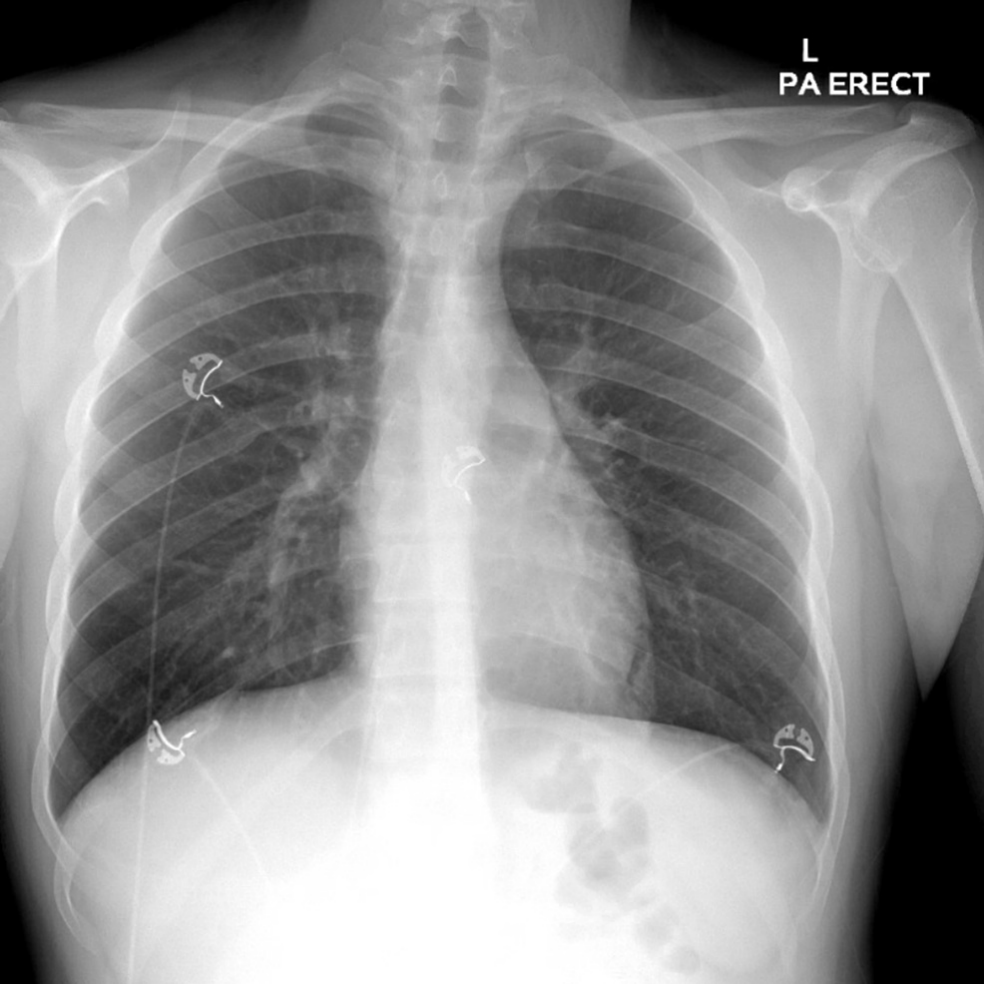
Chest x-ray showing improvement in pneumomediastinum and subcutaneous emphysema L - left; PA - posteroanterior
